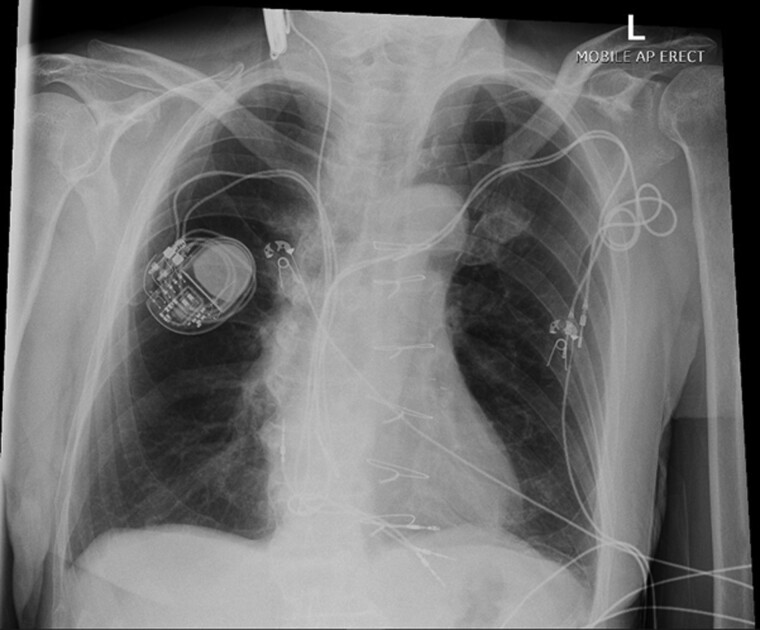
Chest X-ray following external pacemaker implantation demonstrating the presence of five right-sided leads (two leads from the left-sided device, two leads from the right-sided device, and one lead from the external pacemaker).
